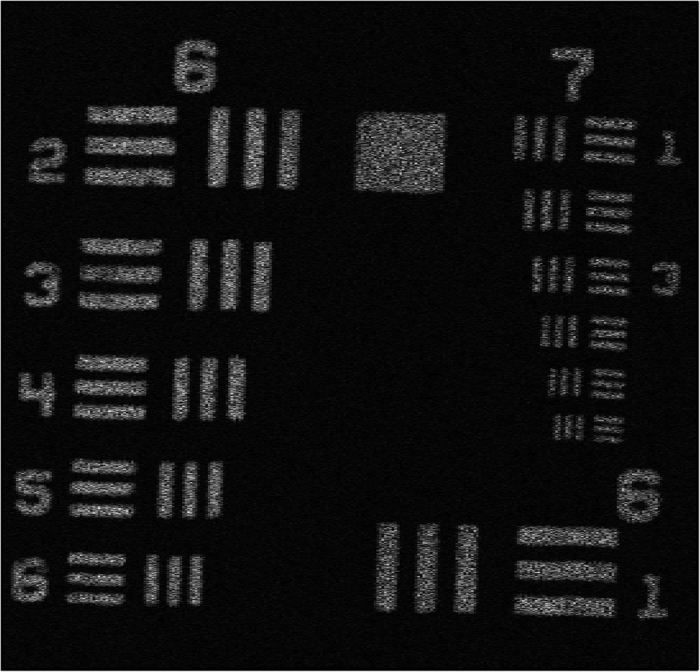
OR-PAM image of a resolution test target obtained by using a fiber laser ultrasound sensor.
